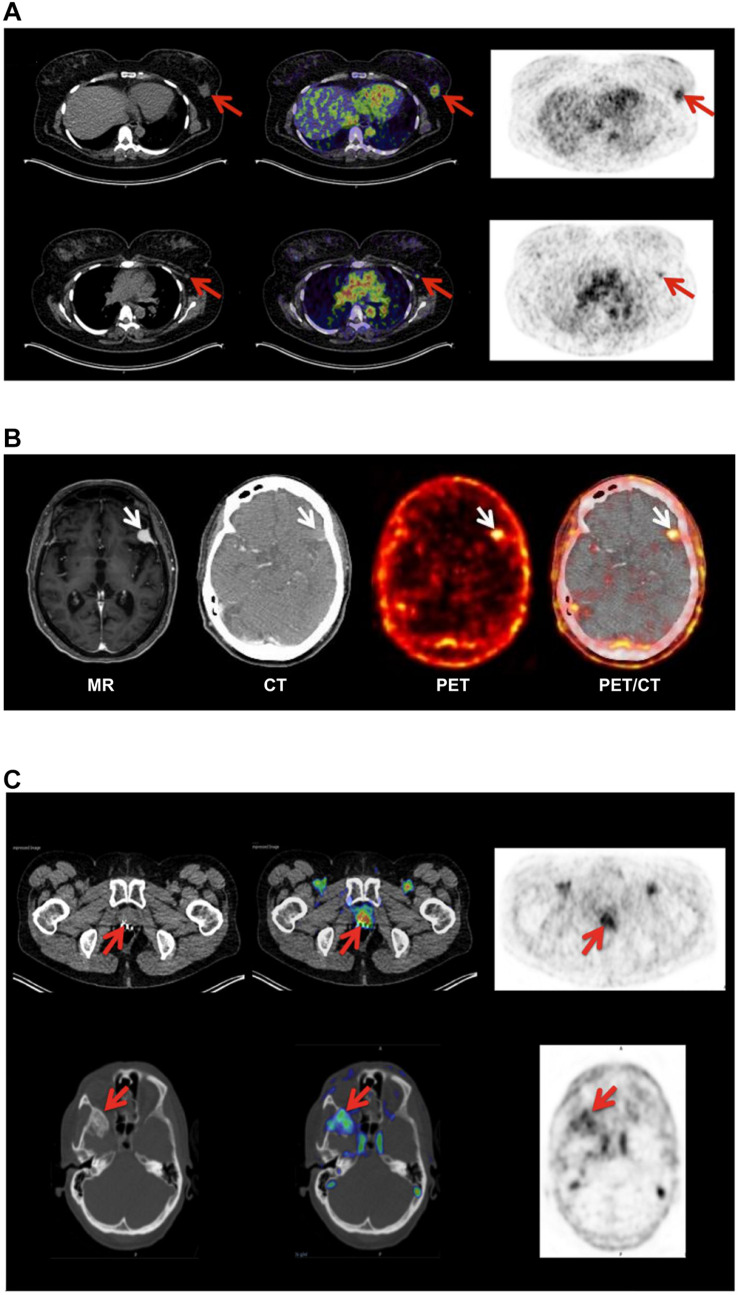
Non-invasive PET imaging of uPAR expression with AE105 as targeting principle. (A) Non-invasive PET/CT imaging of a breast cancer patient 10–60 min post *iv* injection of ^68^Ga-NOTA–AE105. Shown to the left are the CT scans, to the right PET scans, and in the middle the merged images. The red arrows highlight (i) the uPAR-positive primary breast cancer lesion (upper row) and (ii) an uPAR-positive axillary lymph node lesion (lower row) that escaped detection by preoperative ultrasound scanning and fine-needle biopsy. (B) Non-invasive PET/CT image of the transversal cross-section of the brain of another breast cancer patient with confirmed primary breast cancer lesion 60 min post *iv* injection of ^64^Cu-DOTA–AE105. High tracer uptake was unexpectedly noted in a brain lesion that was later confirmed by magnetic resonance scanning (MR) and diagnosed as a meningioma (white arrow). (C) PET/CT imaging of a prostate cancer patient using ^68^Ga-NOTA–AE105 to visualize uPAR expression. Red arrows highlight tracer accumulation in the primary prostate lesion (upper row) and in a metastasis lodged in the sphenoid bone (lower row). Images were reproduced from Persson et al. (2015) and Skovgaard et al. (2017) under the CC-BY license.
